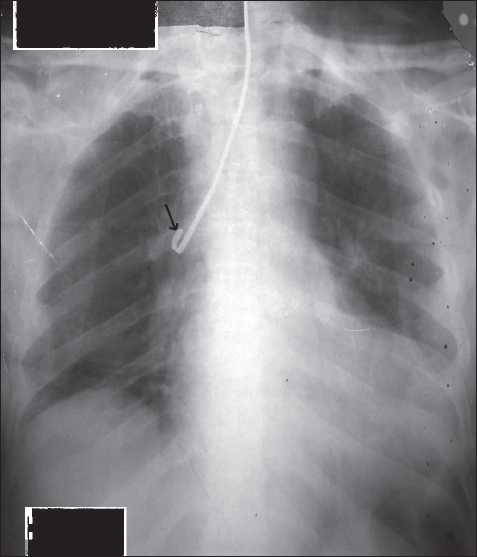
X-ray with metallic tongue cleaner in the airway through tracheostoma. Arrow - Curved distal stuck end
